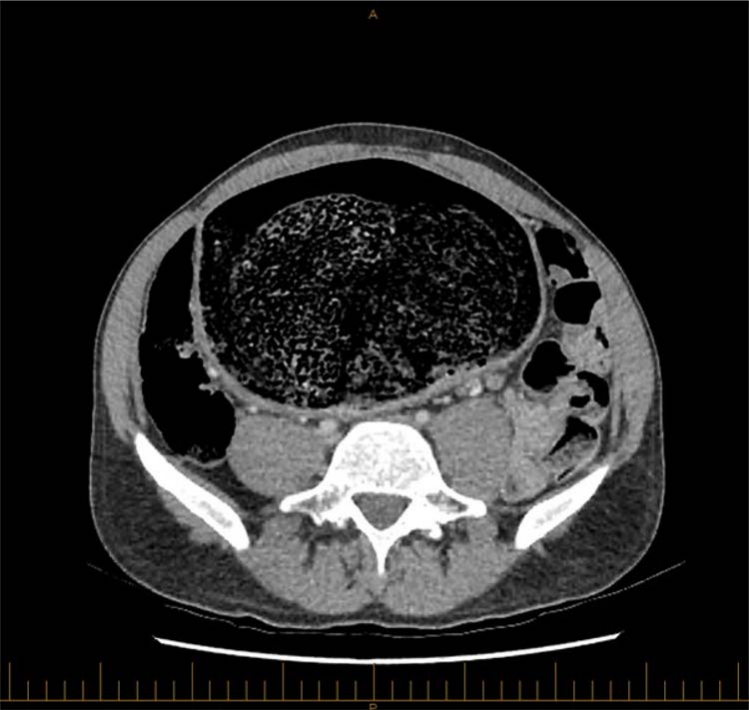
Axial view of CT image of abdomen.
